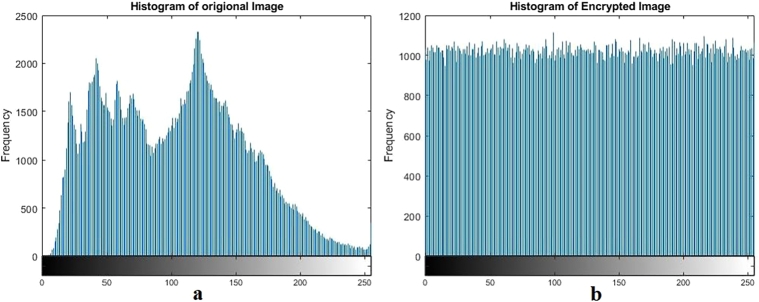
Histogram of plain and encrypted ultrasound image.
